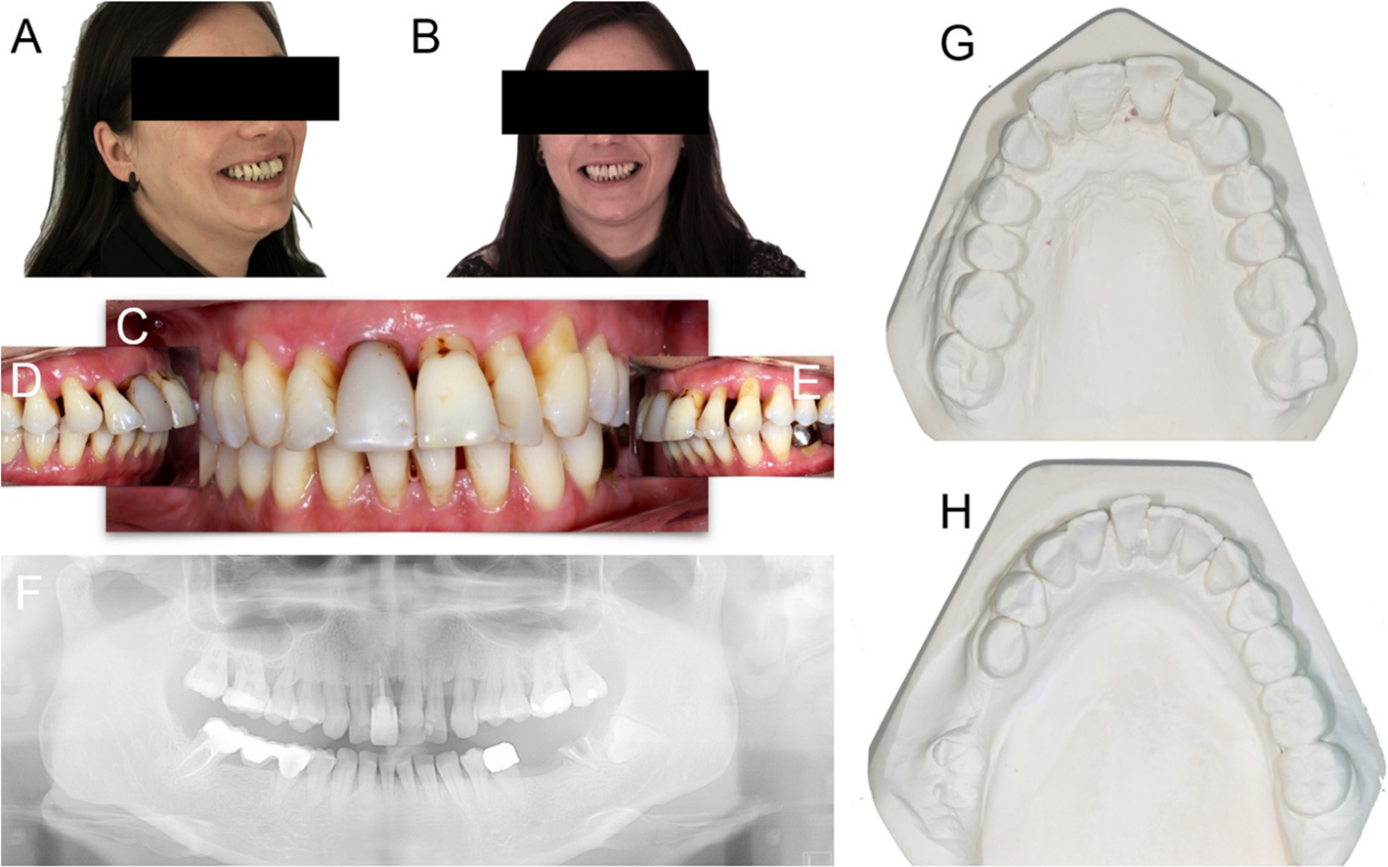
Pre-operative initial situation. Lateral (a) and frontal (b) view of a 32-year-old female patient, in good systemic health, presented with an advanced chronic periodontitis, teeth mobility and recurrent infections, persisting over years. In the upper jaw, in particular, all teeth were sensitive and elongated and this seriously compromised the function and the aesthetic of the smile. The patient suffered from this situation and she was strongly motivated to solve this biological, functional and aesthetic problem. c Frontal and (d, e) lateral intraoral pictures. f Panoramic radiograph. In the maxilla, the severe chronic periodontitis determined an advanced bone loss, that compromised the stability of all teeth. g Upper and (h) lower jaw precision models
